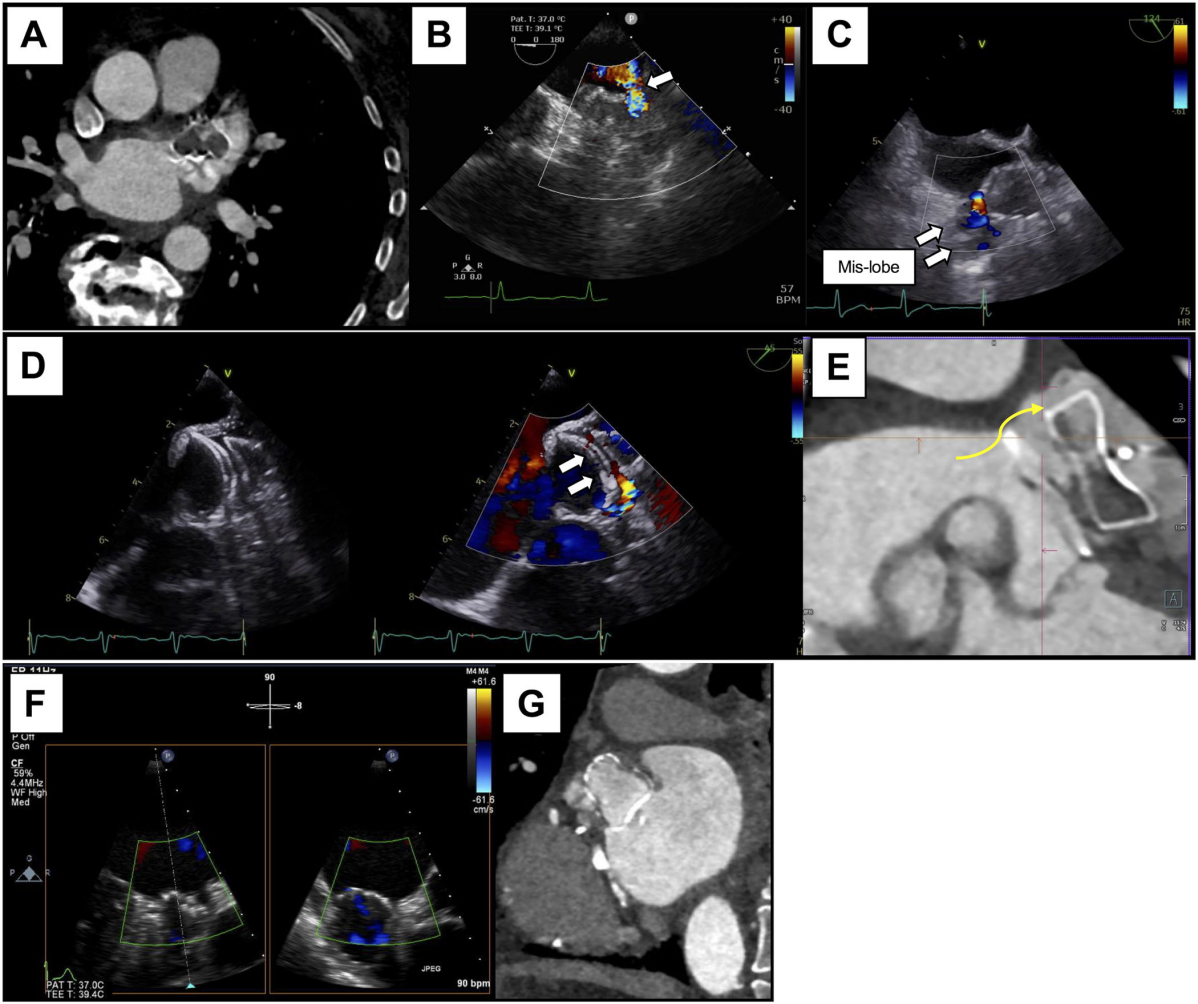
Typical images of residual leaks related to the device. (A) Off‐axis, (B) leak from the ridge side, (C) leak due to mis‐lobed, (D) leak due to disk distortion, (E) leak to the distal disk. Fabric leaks from the Watchman device can be observed in (F) transesophageal echocardiography and (G) computed tomography.
